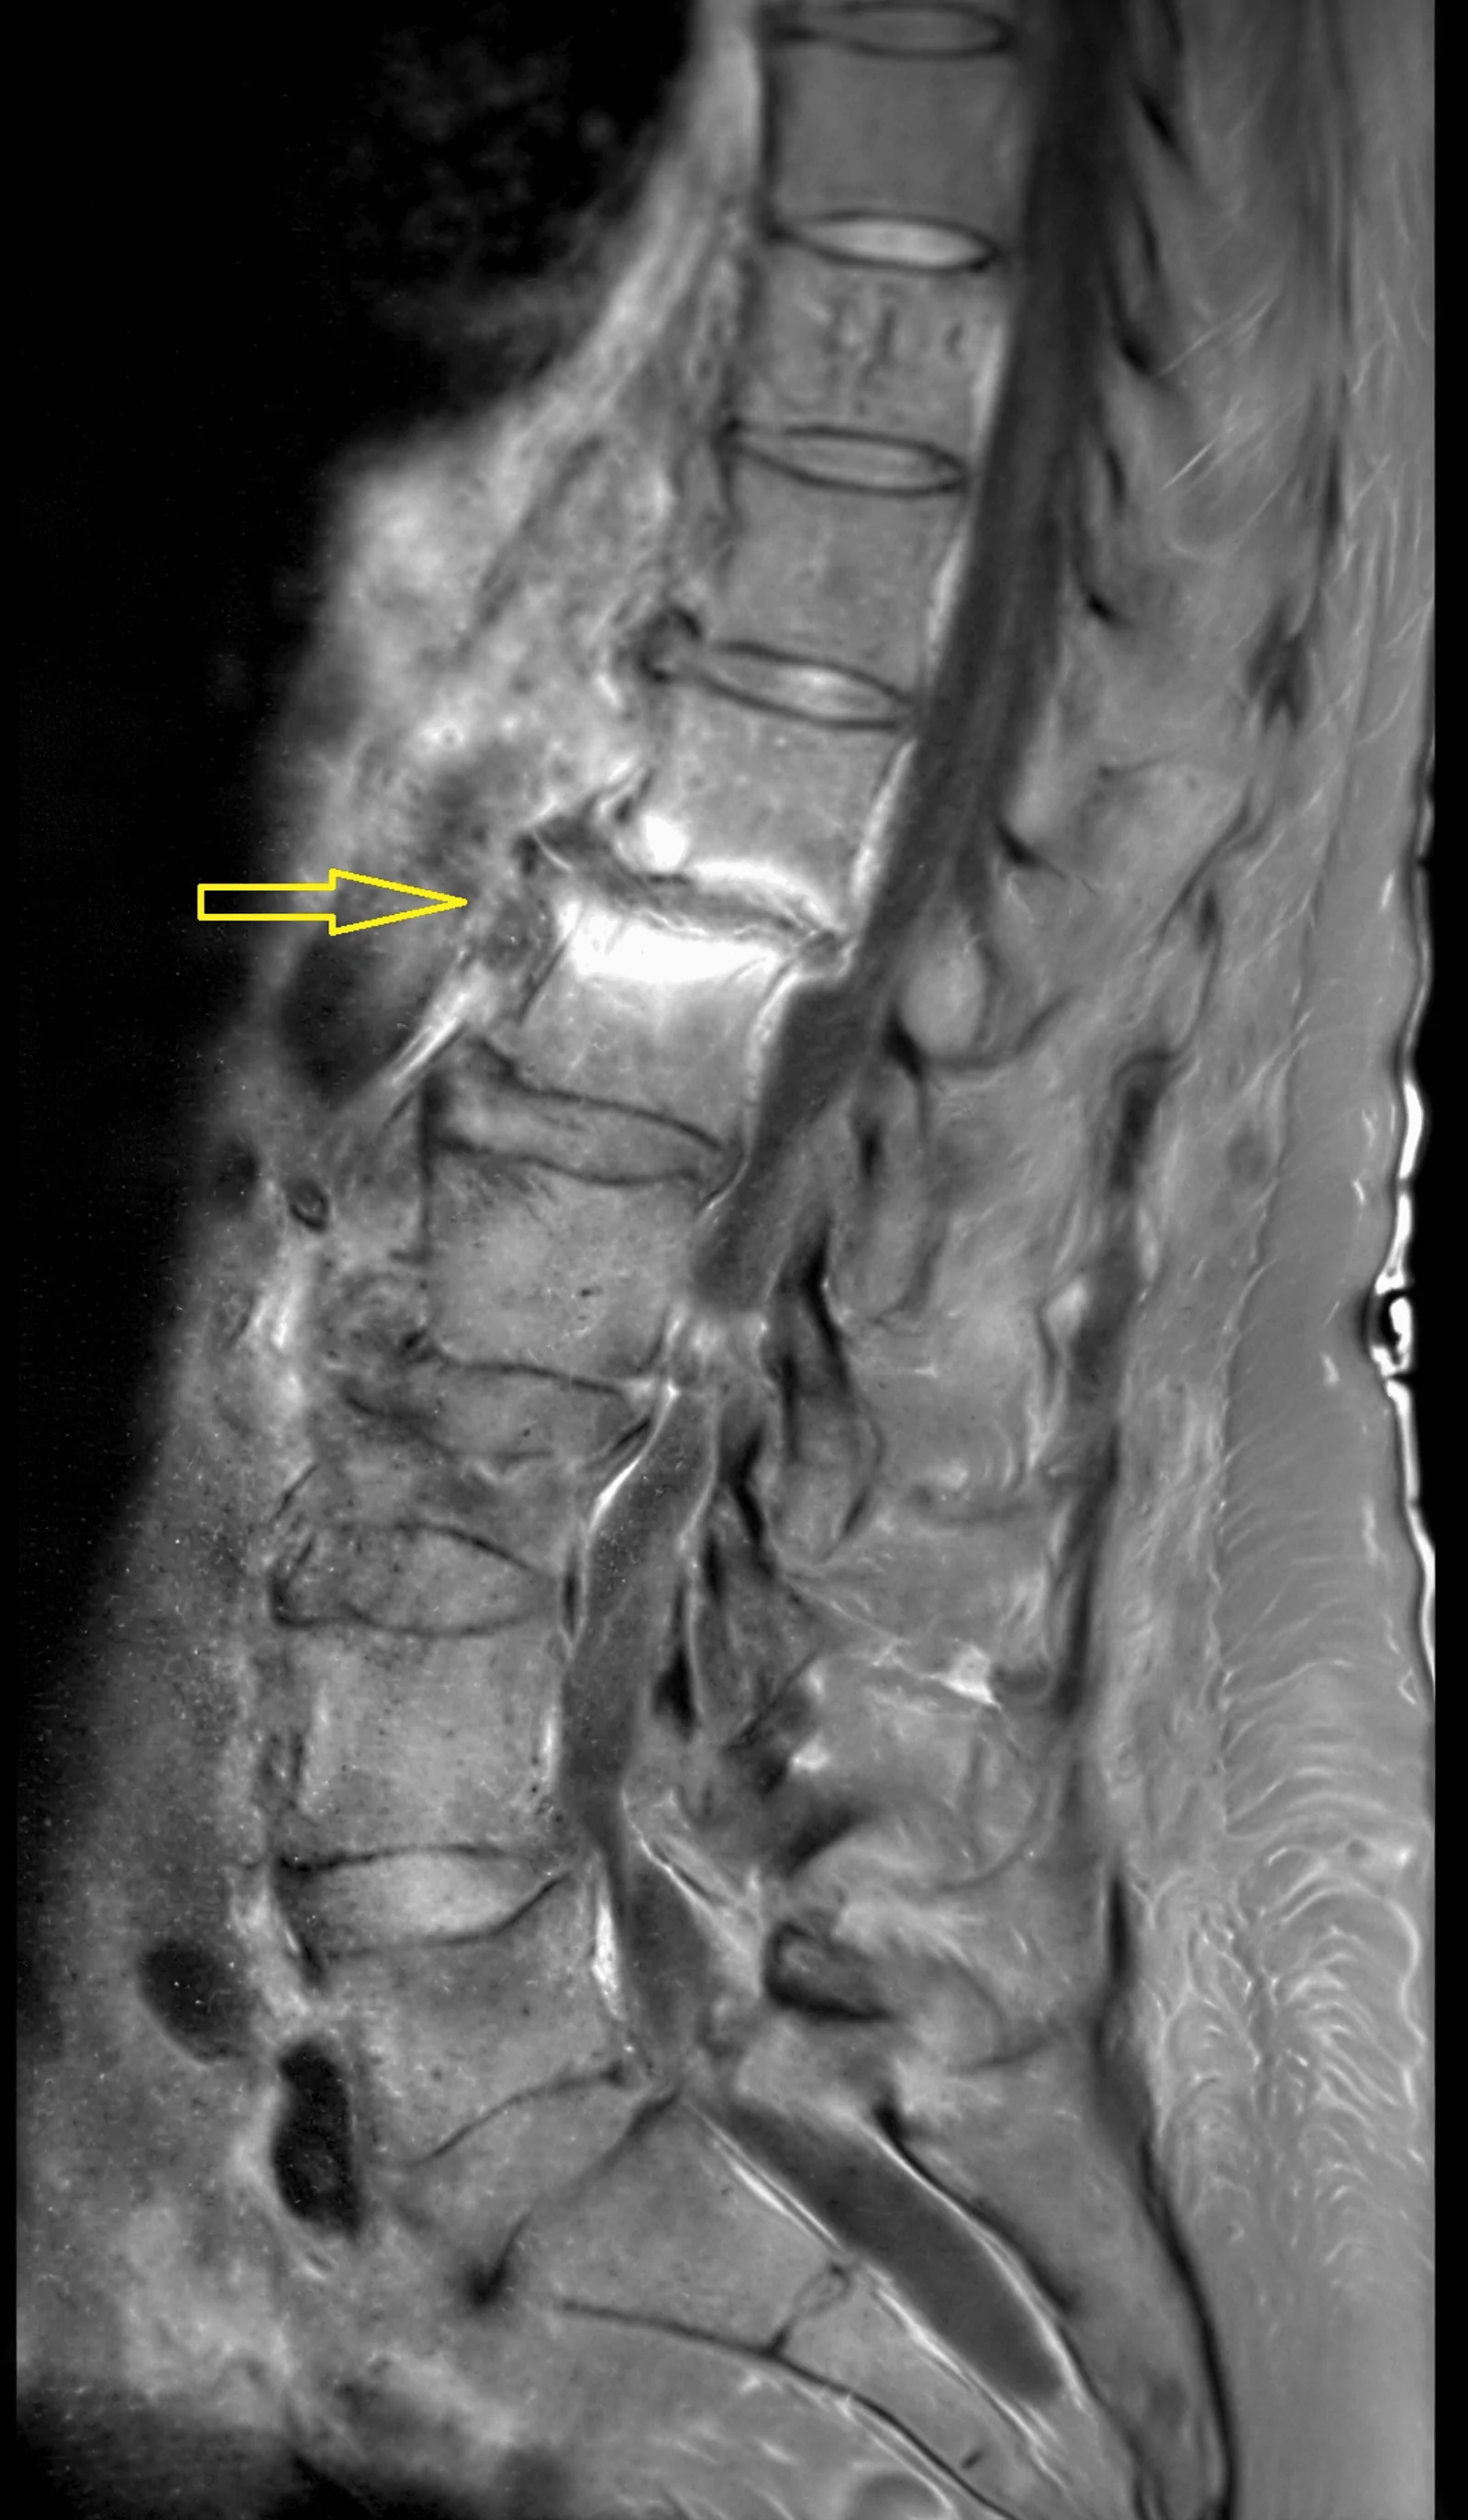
MRI lumbosacral spine with contrast High signal intensity with the adjacent T12-L1 endplate is consistent with interval development of discitis and osteomyelitis (yellow arrow)
